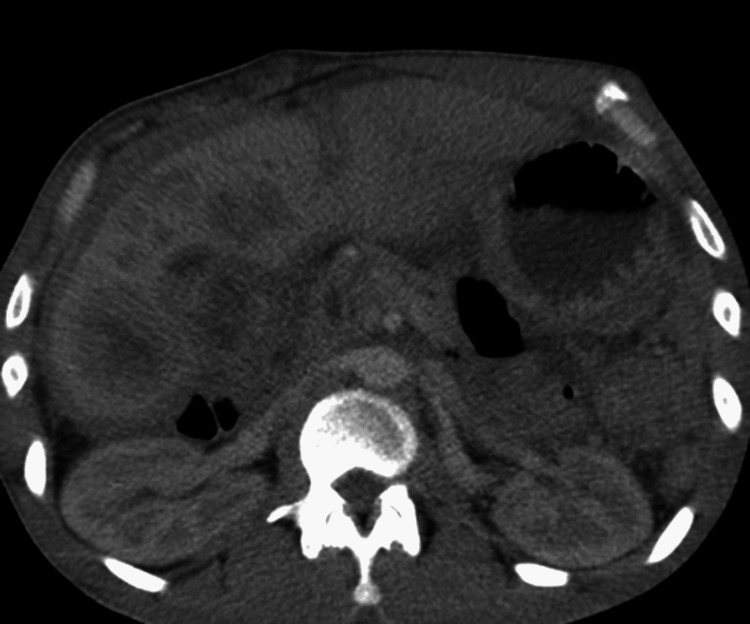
CT of the abdomen and pelvis demonstrating gallbladder mass extending into the lower margin of the liver and invading the duodenum, right hepatic flexure, and pancreatic head; extensive peritoneal carcinomatosis.
